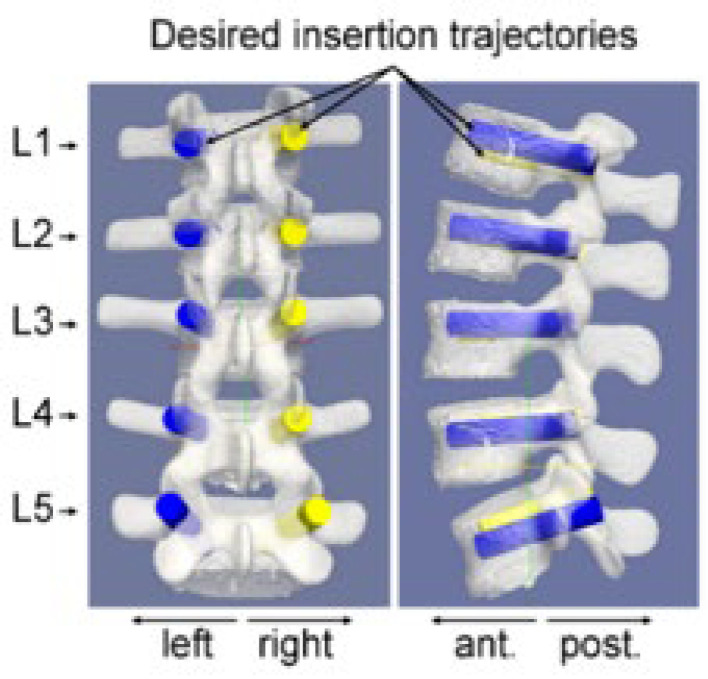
Preoperative planning of the screw insertion on the 3D CBCT model of the lumbar spine. The planning consists of ten desired insertion trajectories, including left and right pedicle screws within each of the five lumbar vertebrae (L1, L2, L3, L4 and L5). The coordinates of the entry and target points of the ten desired screw positioning are stated in the reference frame R0 of the CBCT images.
